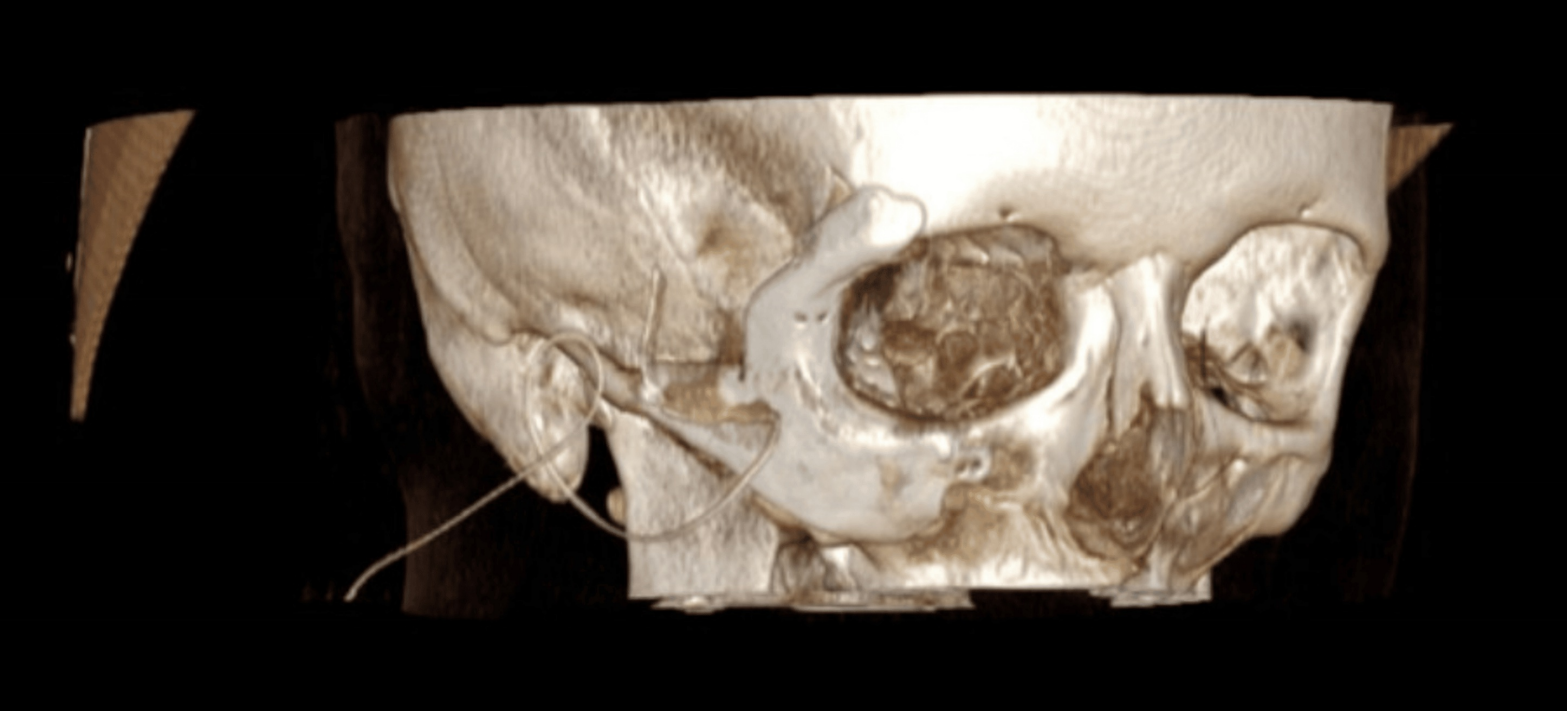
3D right-sided view of CT head with right orbito-zygomatic implant
